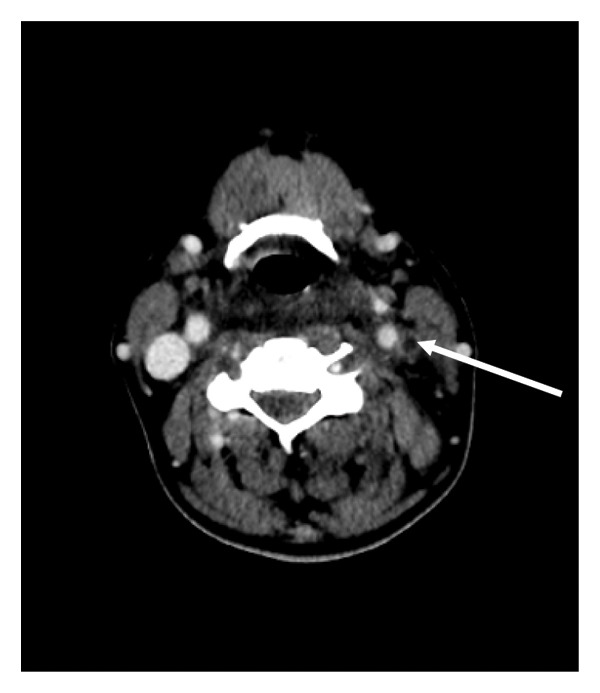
Transverse CT scan. Left internal jugular vein thrombosis.
